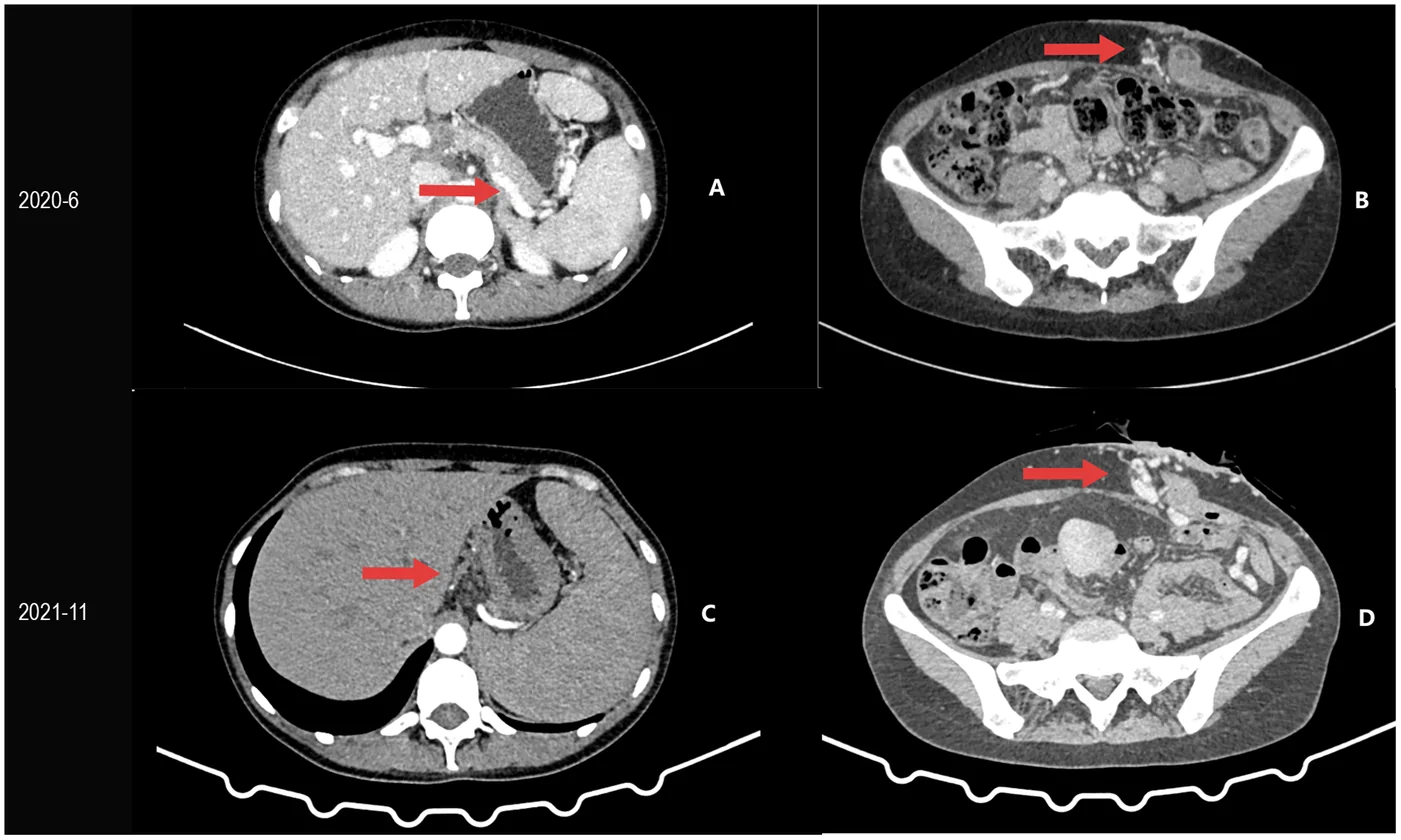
Contrast-enhanced CT demonstrating features of portal hypertension, including gastroesophageal varices and tortuous peristomal collateral vessels. (A) The red arrow indicates tortuous and dilated splenic vessels; (B) The red arrow indicates tortuous peristomal collateral veins; (C) The red arrow indicates dilated gastroesophageal varices adjacent to the gastric fundus; (D) The red arrow indicates progression of the peristomal collateral veins compared with the previous examination.
